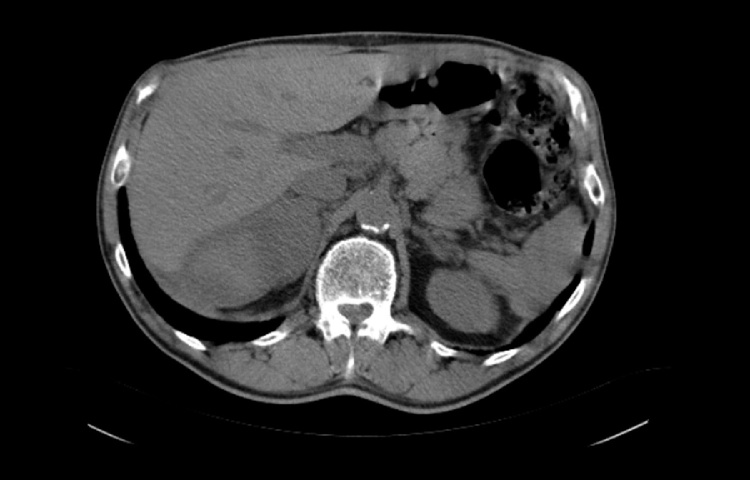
Abdominal CT scan demonstrating a large retroperitoneal mass that was ultimately identified as a metastasis due to lung cancer.
